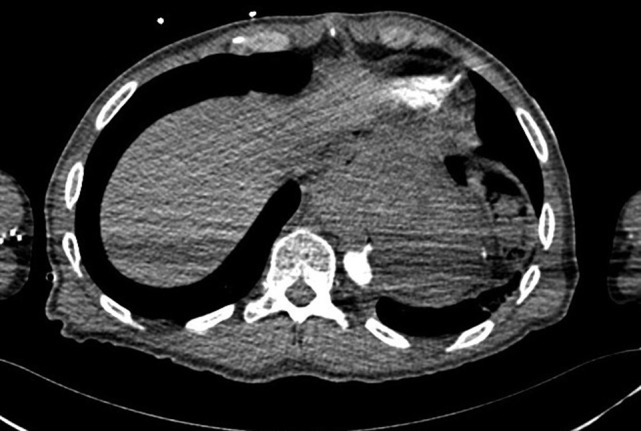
CT aortogram showing the extravasation of contrast into the stomach.
